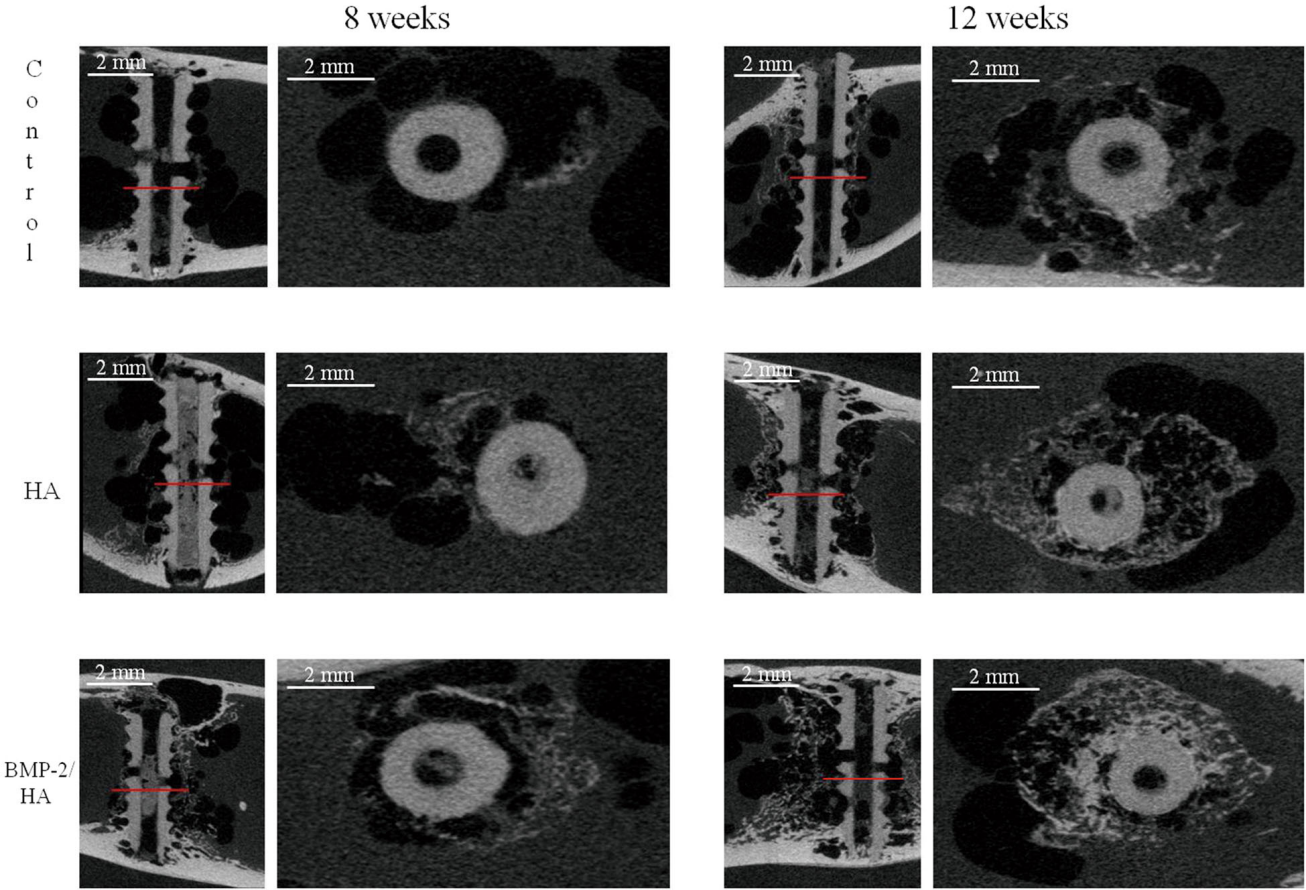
Coronal and sagittal plane micro-computed tomography images at 8 and 12 weeks postoperative. Red lines indicate the position of the sagittal plane from the coronal plane of the screw
